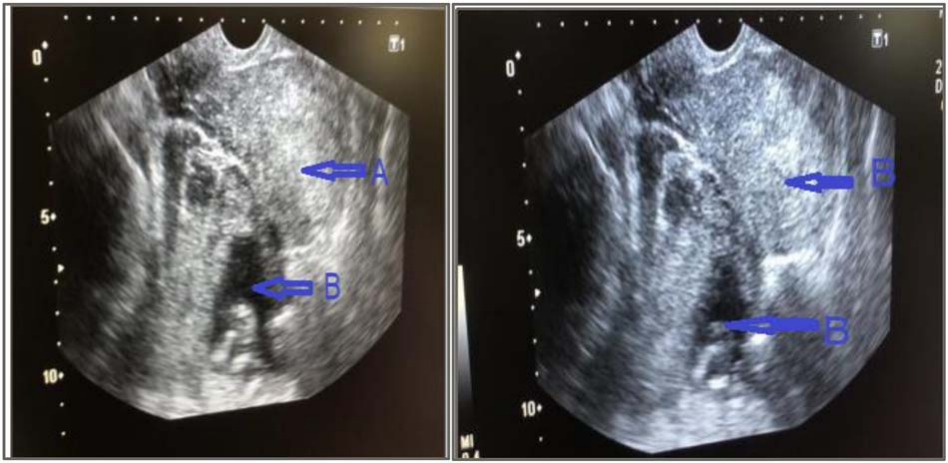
Trans vaginal ultrasound was done that showed, (a) empty uterine cavity, with no gestational sac, (b) live fetus of 14 weeks 6 days in left adnexa and moderate amount of free fluid in pelvis.
